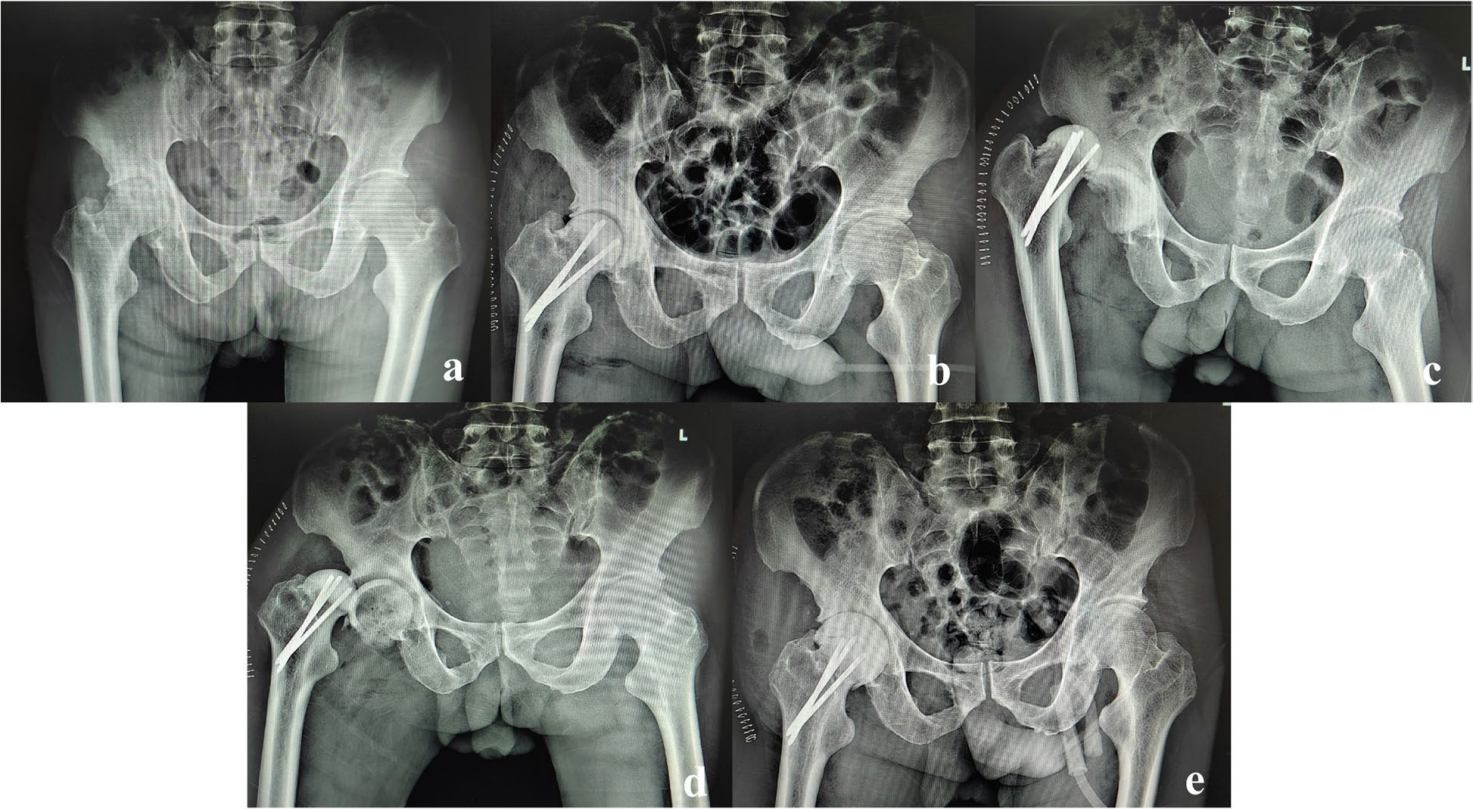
One case of antibiotic-load temporary spacer migration. A 73- year-old male patient was diagnosed with right hip purulent infection and underwent debridement and antibiotic-loaded bone cement temporary spacers. PD occurred 3 days after the operation, and the Allis maneuver was applied for reduction. Spacer migration was confirmed from the AP view of the pelvic X-ray film, and open reduction with spacer exchange was performed. a. Preoperative X-ray film of the pelvis. b. AP view X-ray film of the pelvis immediately after the operation. c. AP view X-ray film of the pelvis, showing PD occurred. d. AP view X-ray film of the pelvis immediately after closed reduction with the Allis maneuver, showing spacer migration. e. AP view X-ray film of the pelvis immediately after open reduction and spacer exchange operation
